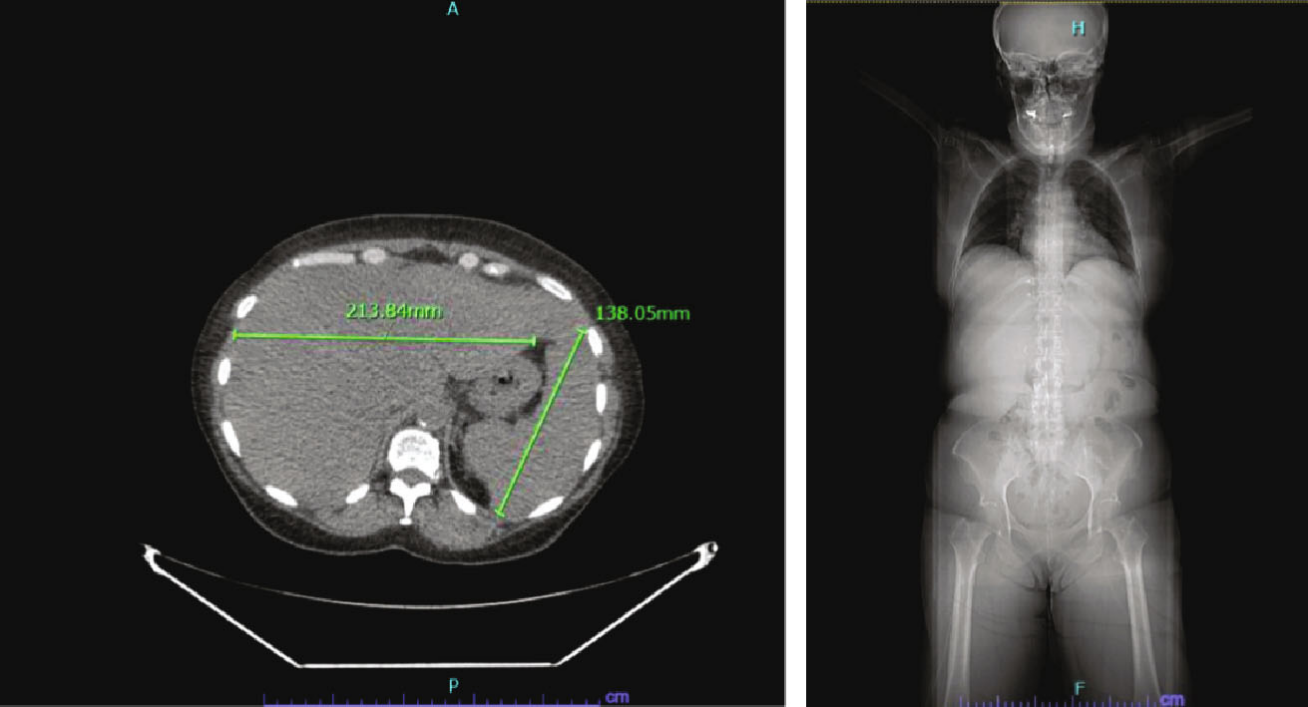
CT scan skull base to midthigh findings. Axial view (a): the spleen and liver borderline enlarged. Coronal view (b): no focal abnormalmality in the distal esophagus and stomach with no masses, adenopathy, or further evidence of regional or distal metastatic disease.
